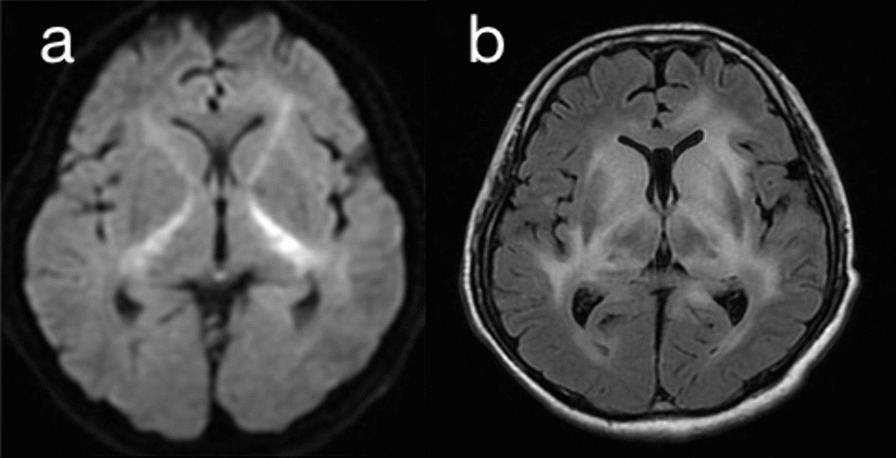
Head magnetic resonance imaging on admission day. a With diffusion-weighted imaging. b With fluid-attenuated inversion recovery imaging
